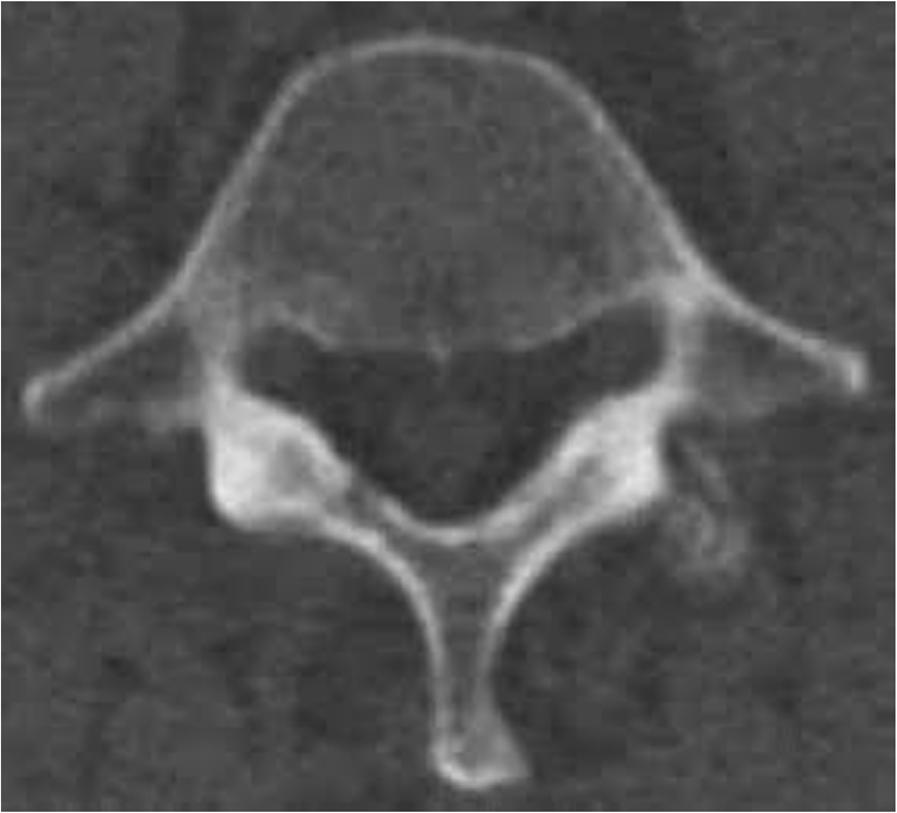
CT bone window image of L4–5 which does not clearly demonstrate evidence of spinal epidural lipomatosis
